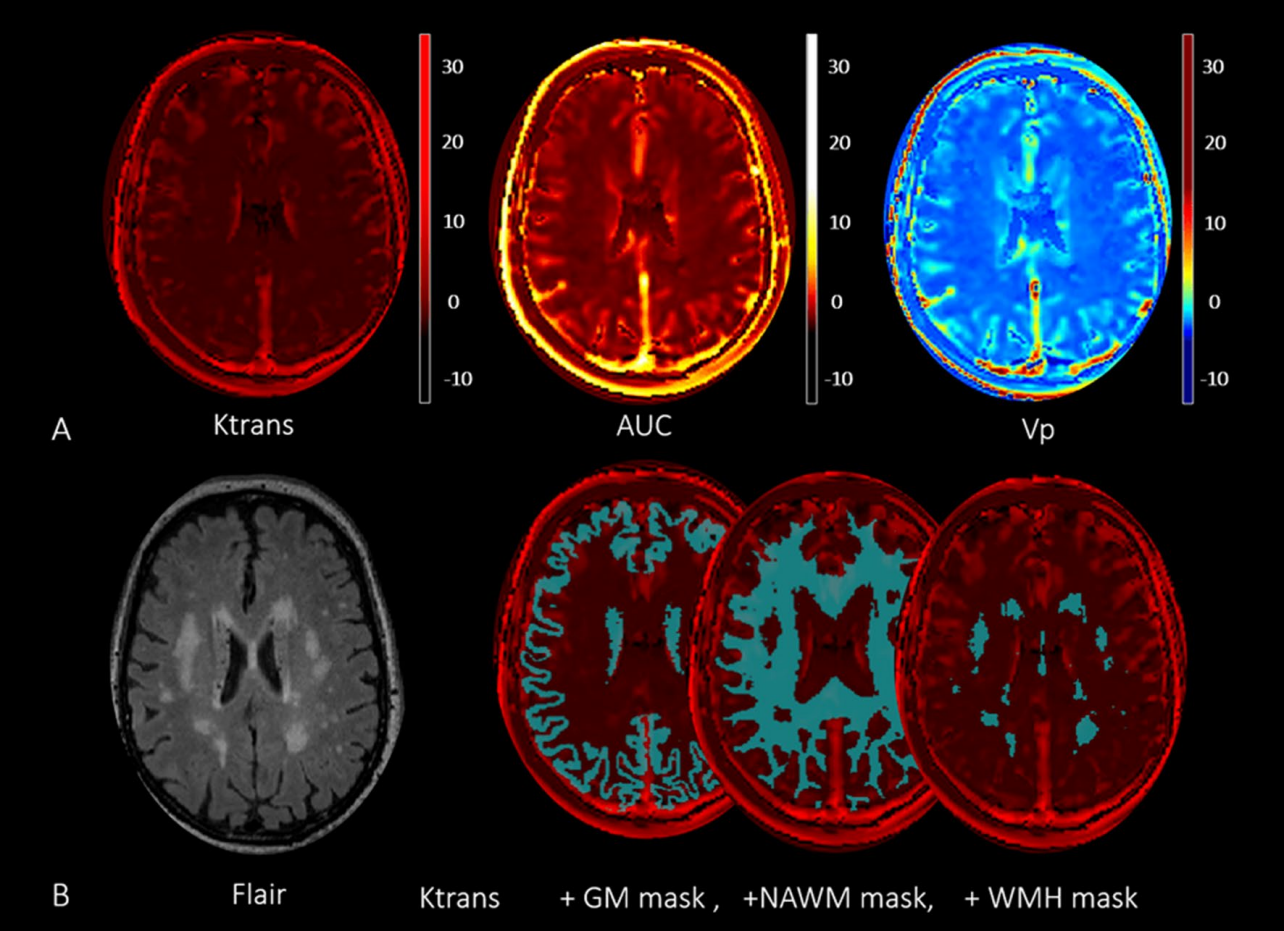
Example of MRI data of CSVD patient. Ktrans, AUC and Vp maps (A). T2-Flair image and superimposion of GM, NAWM and WMH masks over the individual ktrans permeability map (B).
